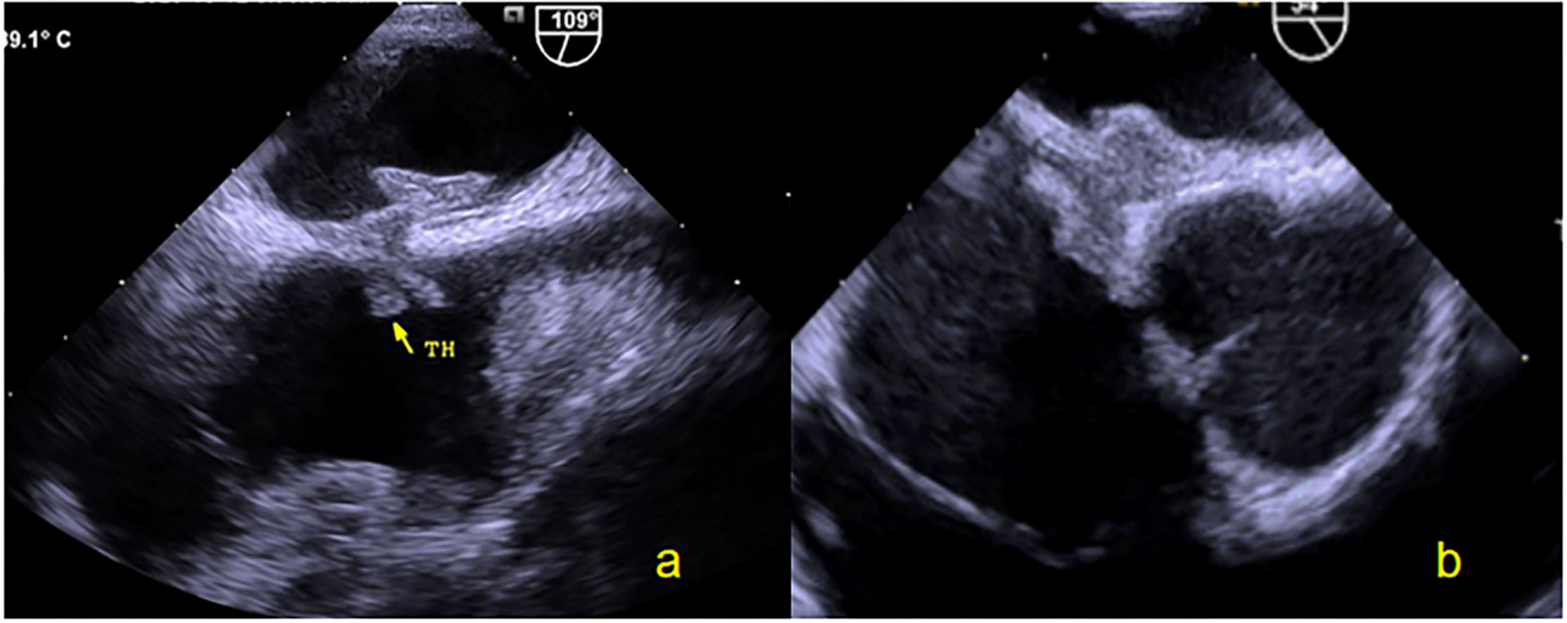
Thrombus observed by TEE. (a) Thrombus was found on the surface of the right occluder device by two-dimensional TEE at 6 months. (b) Thrombus of the right occluder device disappeared after 1 month. TEE, Transesophageal echocardiography.
